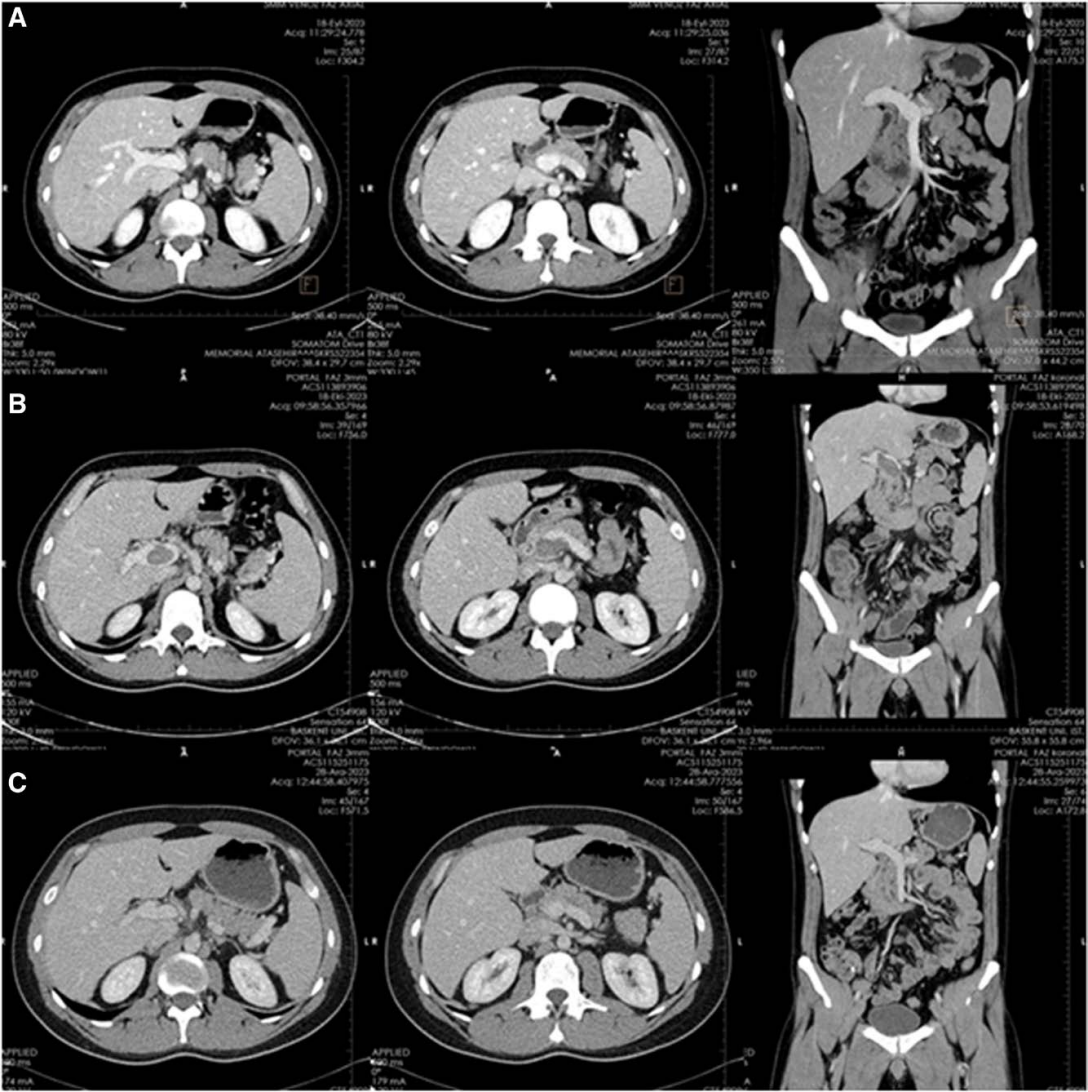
(A) The retrospective analysis of computed tomography (CT) scans performed at the external center during the first emergency service admission of the patient. (B) The CT scans of POD 30. (C) The CT scans of the POD 100.
